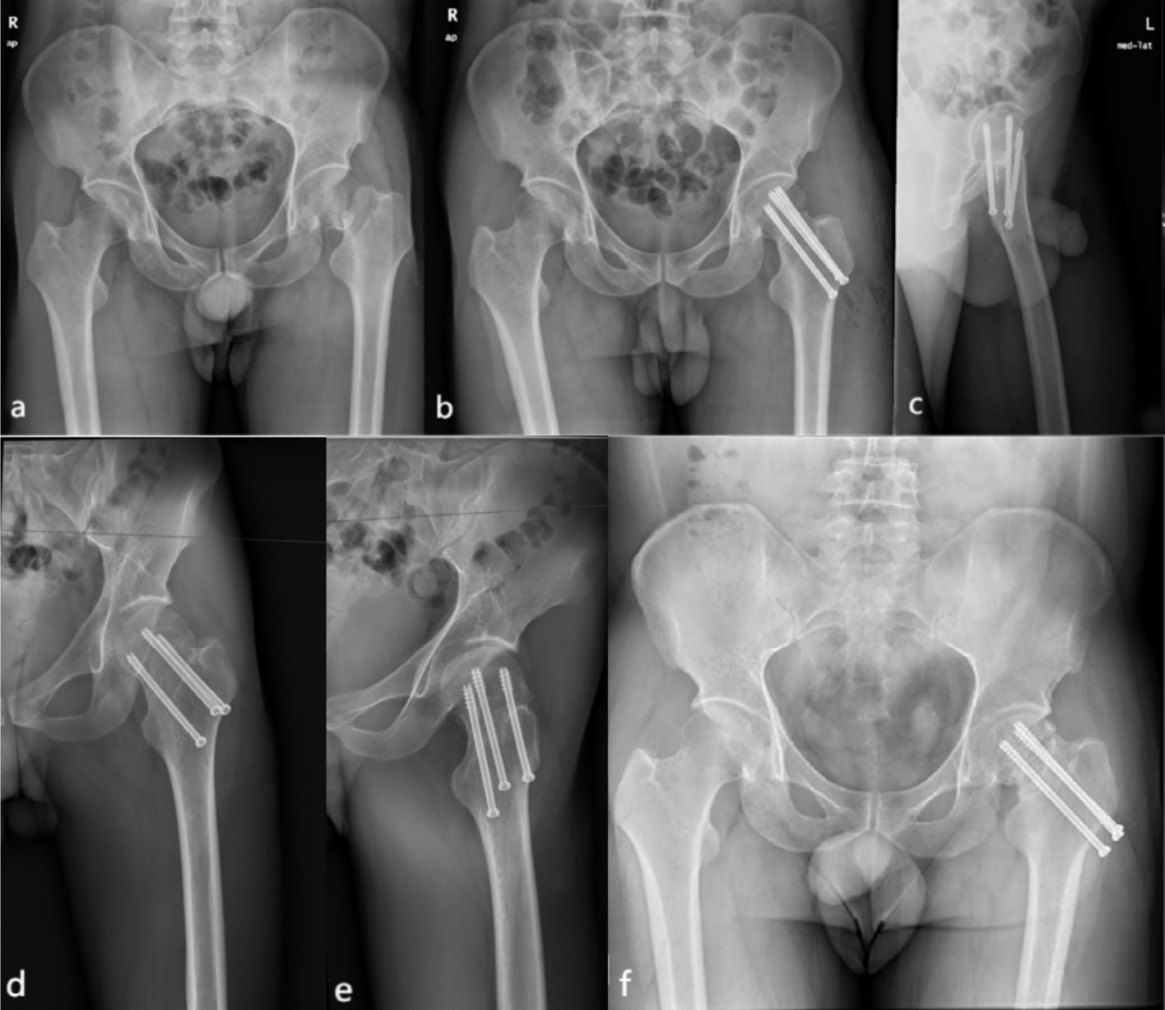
A 47‐year‐old male patient with a left femoral neck fracture was treated with CCS. (a) Preoperative X‐ray image. (b, c) Postoperative x‐ray images. (d, e) Postoperative 6‐month follow‐up radiographs. (f) Postoperative 16‐month follow‐up radiographs showing femoral head necrosis.
